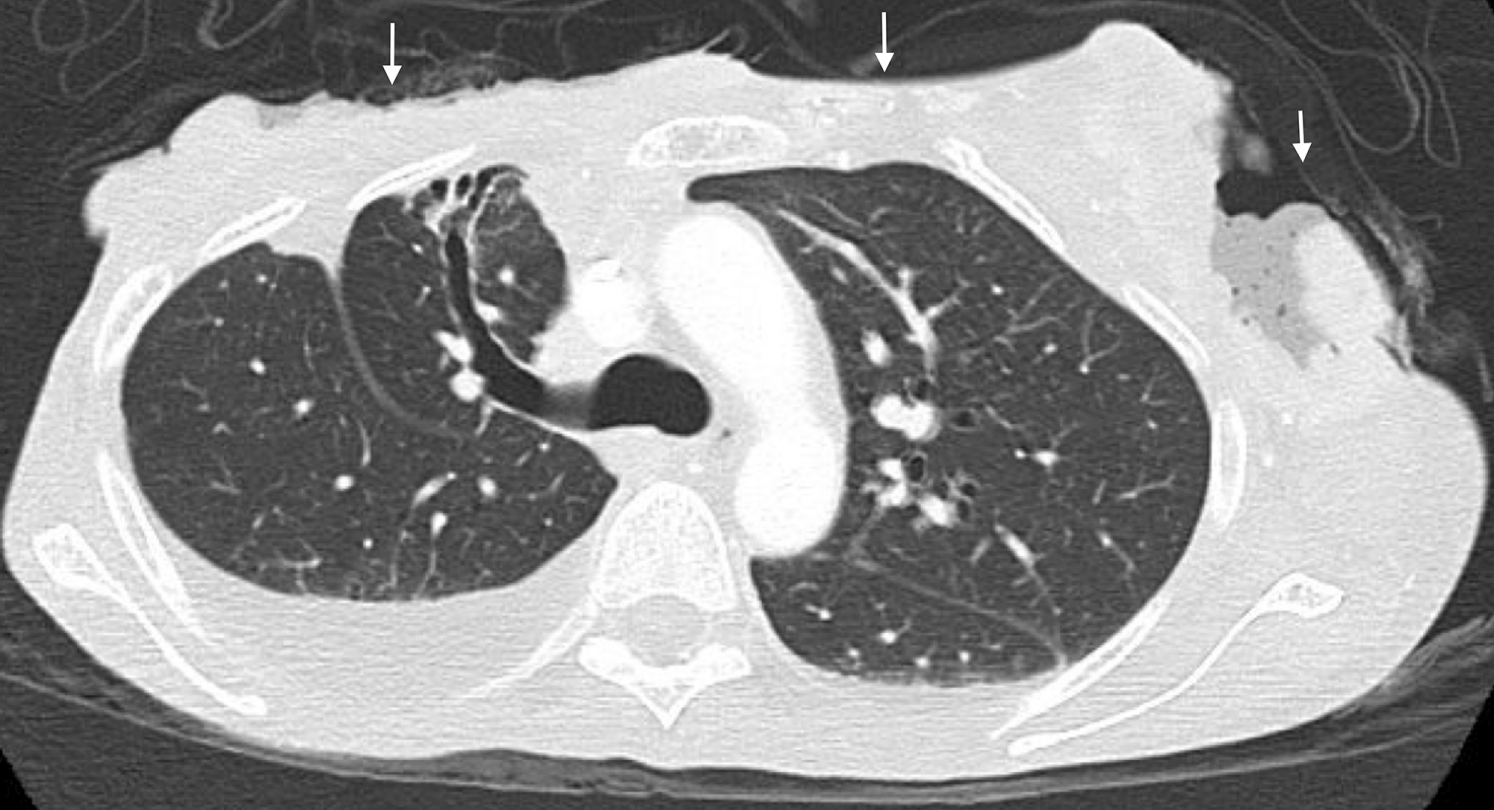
The sagittal cross section from the computed tomography (CT) of the chest that demonstrates the extension of the soft tissue infection at the left lumpectomy and the right mastectomy sites into the sternum and mediastinum.
